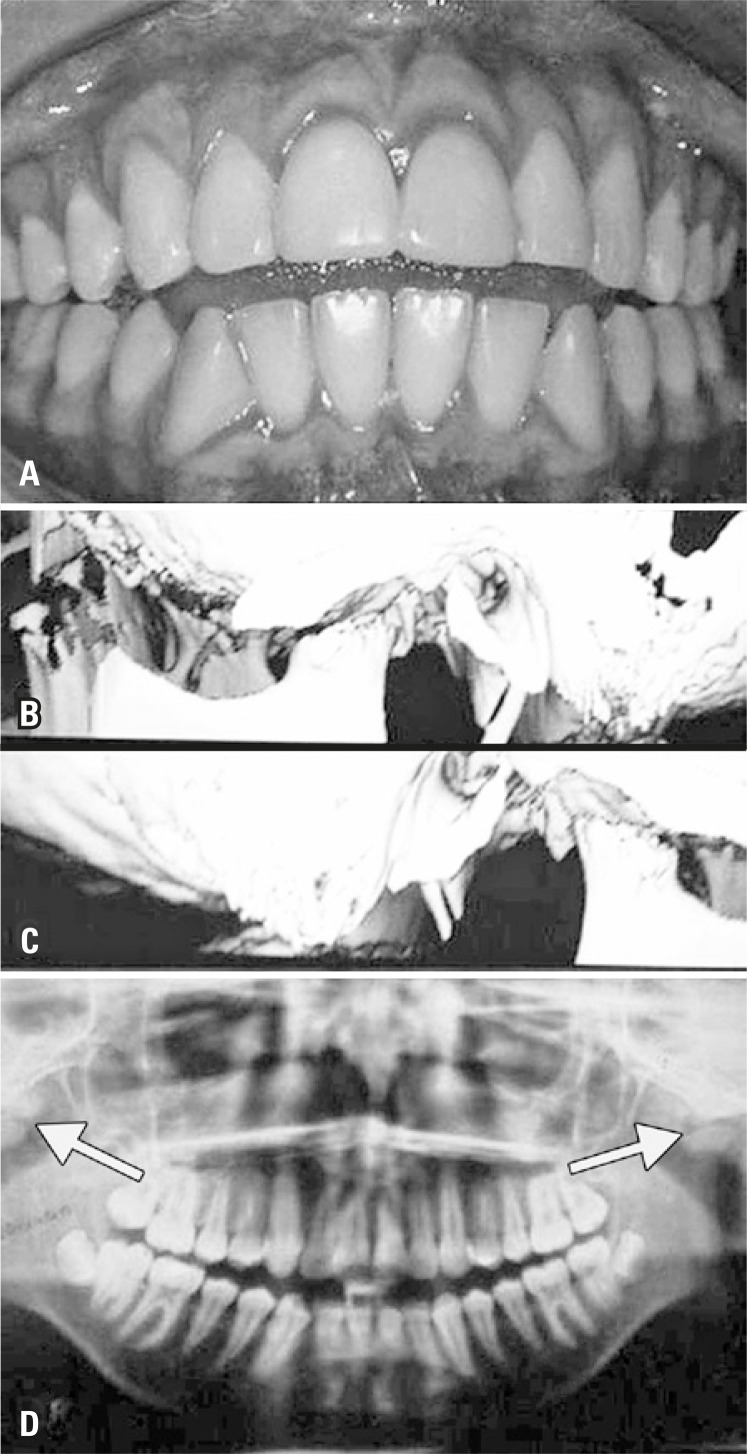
Acute changes in the temporomandibular joints of patient with juvenile idiopathic arthritis, as detected by 3D-computed tomography and panoramic X-ray. The clinical photo of patient show anterior open bite (A). Chronic changes in the temporomandibular joints are shown on sagittal images by three-dimensional computed tomography (B and C), involving condylar flattening, condylar erosions and anterior disk displacement. The same observations were show by panoramic X-ray (D)
